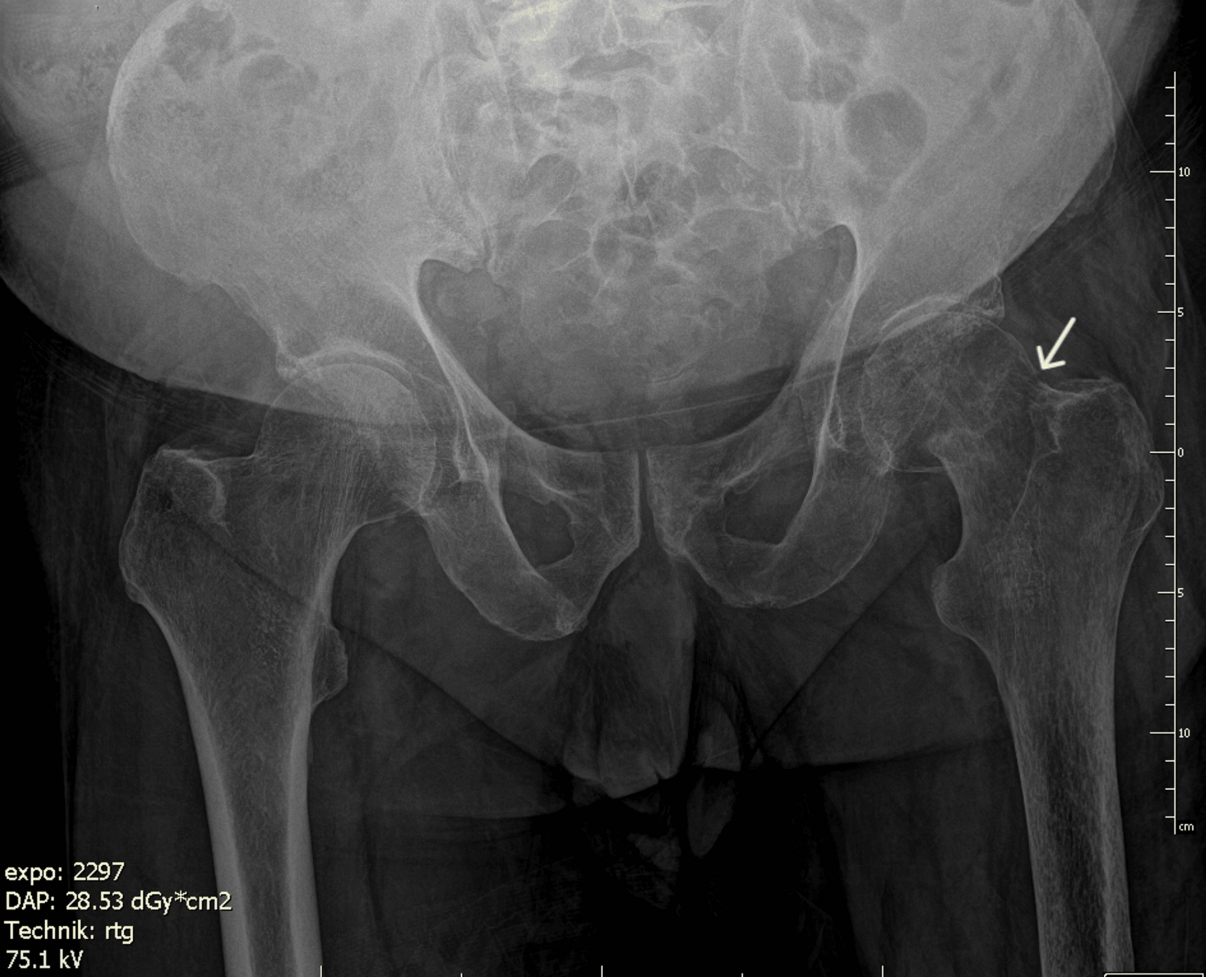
Preoperative AP radiograph showing displaced subcapital femoral neck fracture. The white arrow indicates a subcapital fracture of the femoral neck.
